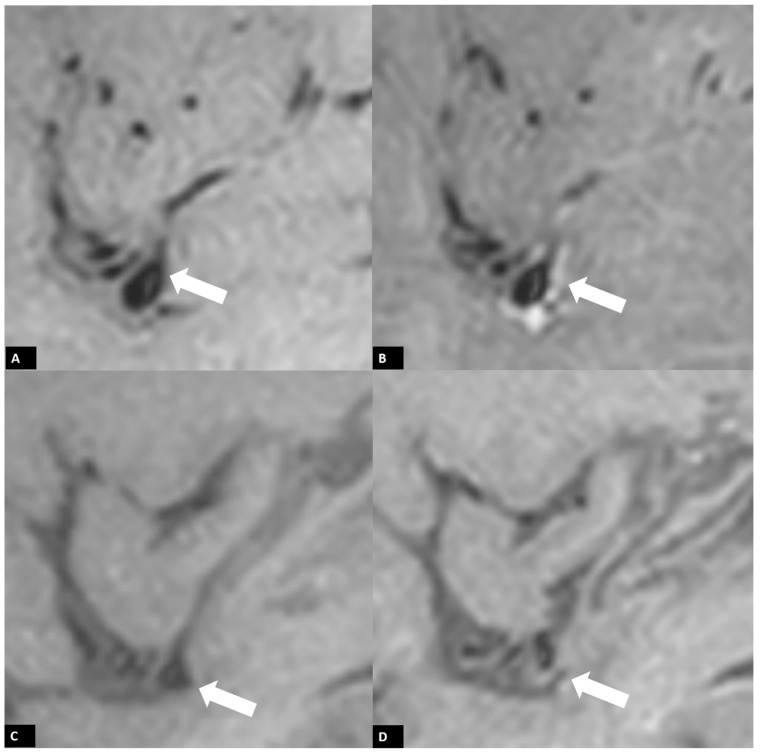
Three-dimensional (3D) black blood T1WI images of vessel wall MRI. A faint high signal on T1WI along the left proximal M2 segment MCA vessel wall due to arterial dissection (arrows) was noted in a follow-up MRI three days after EVT using the pre-contrast sagittal T1WI (A) with eccentric enhancement (B). Follow-up MRI three months after EVT revealed normalization of the vessel wall enhancement (D) and almost normalization of the faint high signal on T1WI (C) in the left proximal M2 segment MCA, indicating that the dissection healed with time.
